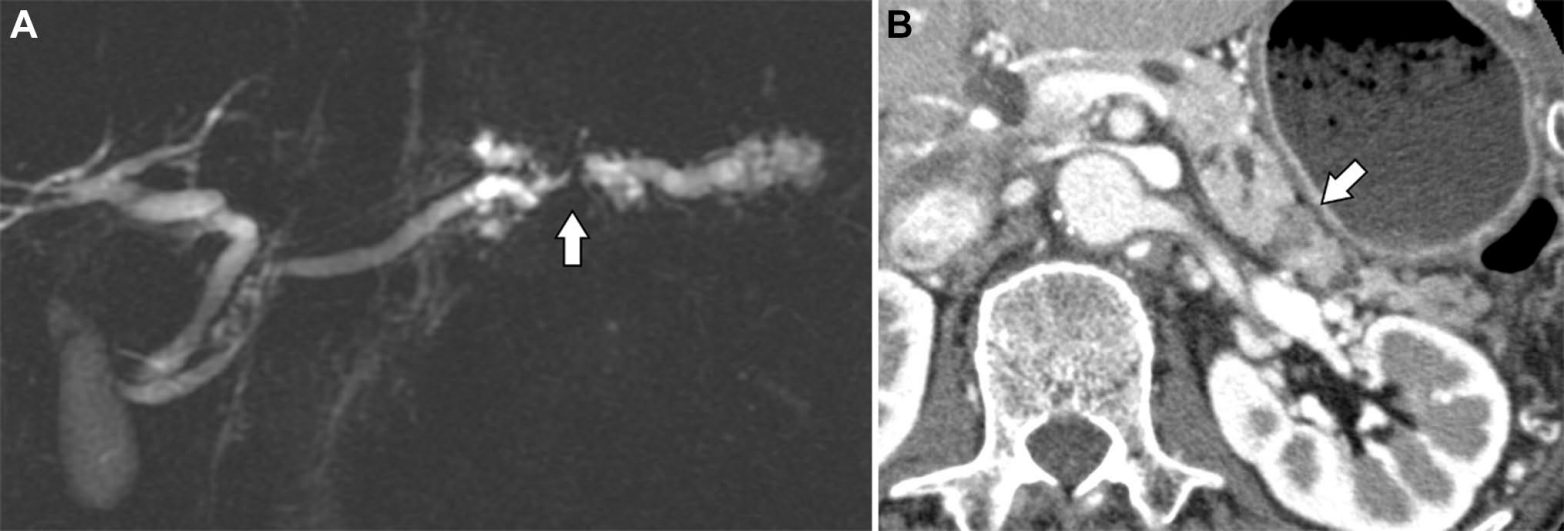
Case of a 70-year-old man under follow-up for intraductal papillary mucinous neoplasm. Magnetic resonance cholangiopancreatography shows obstruction of the main pancreatic duct at the pancreatic tail (a: arrow). Dilatation of the main pancreatic duct downstream of the obstruction suggests mucus production. In the late arterial phase on computed tomography, a hypovascular tumor shows peripancreatic invasion (b: arrow). It was resected and pathologically diagnosed as intraductal papillary mucinous carcinoma with peripancreatic invasion.
